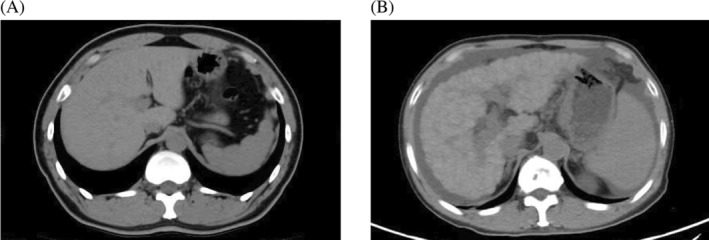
Displays computed tomography (CT) images contrasting healthy individuals (A) with selected cirrhotic patients (B).
